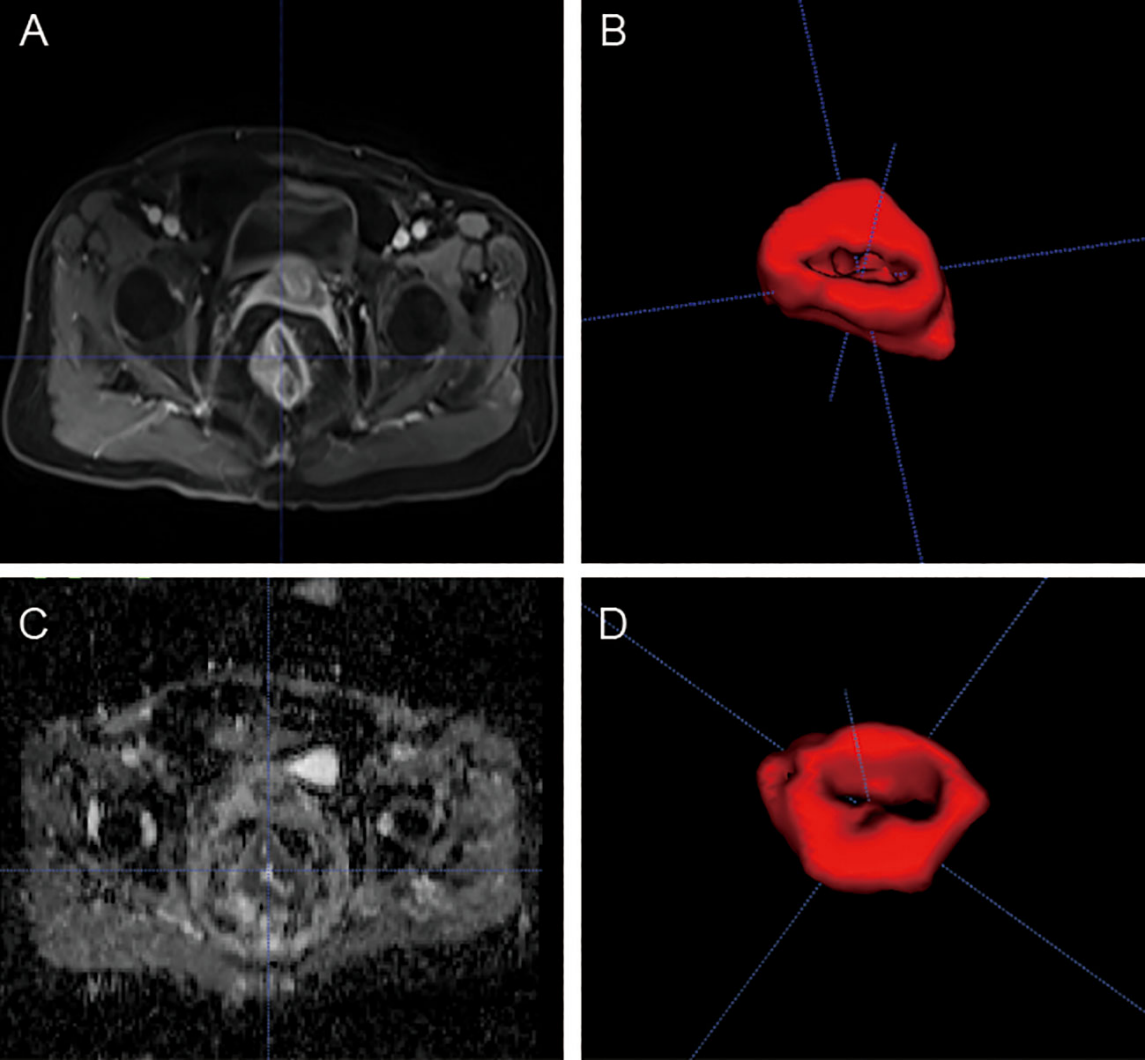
MR images of a 53-yearold man with rectal cancer. Examples of tumors with MRI (A, C) and 3D segmentation (B, D).
